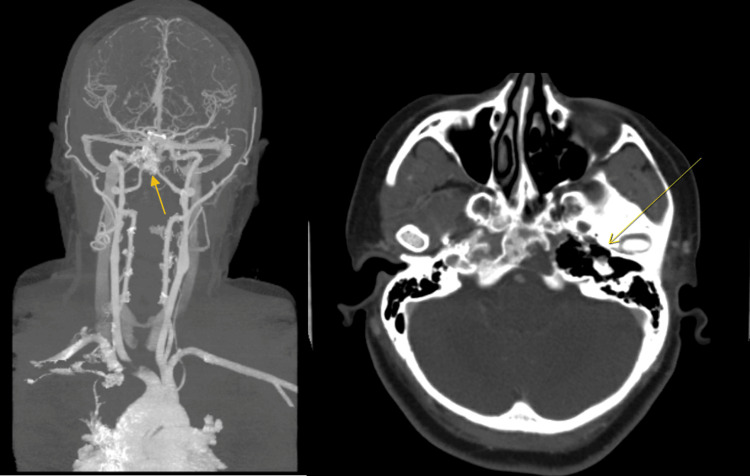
A CT angiogram-processed image shows that the epidermoid cyst at the skull base did not cause a gross invasion or occlusion of the left internal carotid artery. Subsequent imaging demonstrated bone loss at the petrous apex and adjacent clivus.
